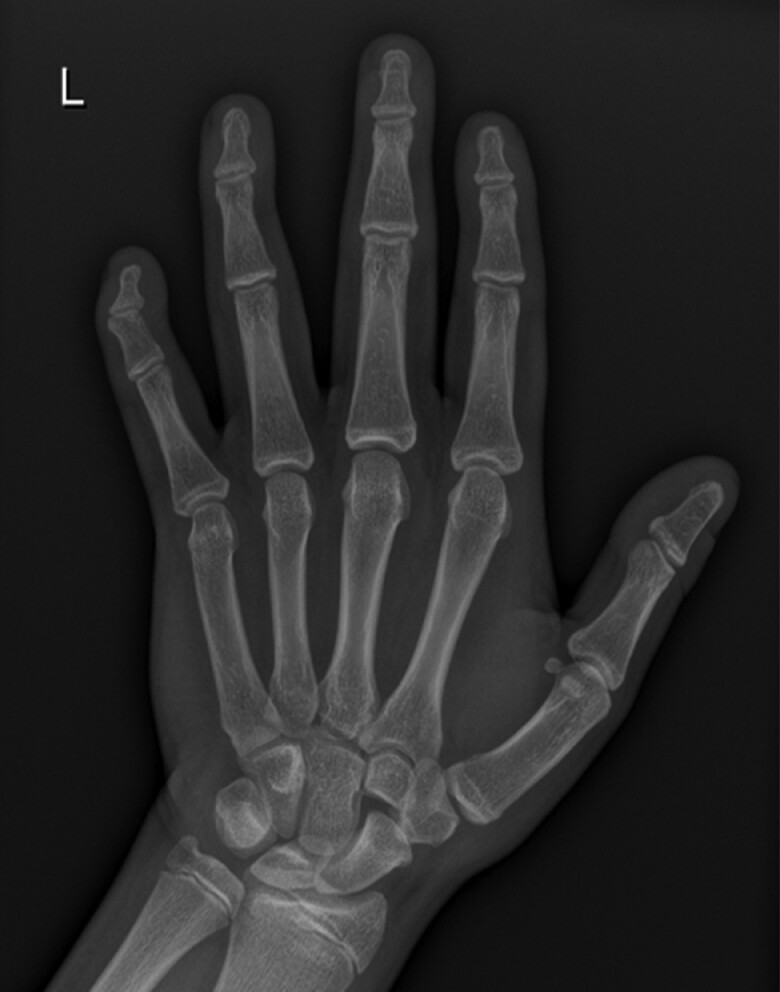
X-ray of the hand and wrist of case 2 showing slightly short metacarpals compared to proximal phalanges; flexion deformity in the distal interphalangeal joint of little finger.
